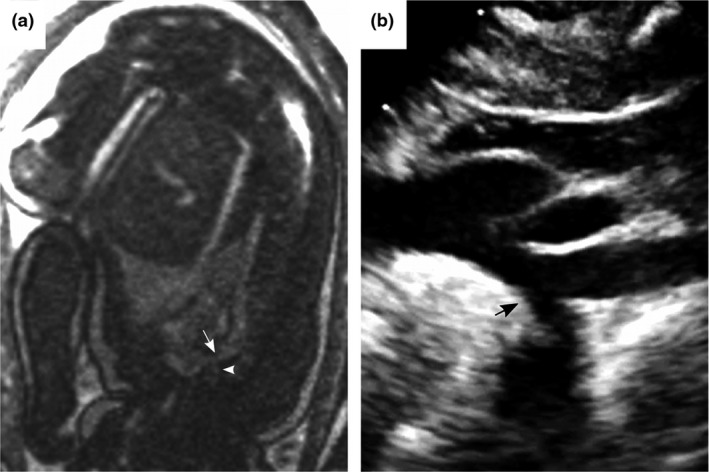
Fetal balanced steady‐state free‐precession (bSSFP) magnetic resonance imaging of the aortic arch (a) showed a hypoplastic aortic arch with minimum diameter 2·8 mm (arrow) in the aortic isthmus. The left subclavian artery is denoted (arrowhead). Images are shown without interpolation. The presence of a hypoplastic arch was confirmed by echocardiography 10 days after delivery (b).
